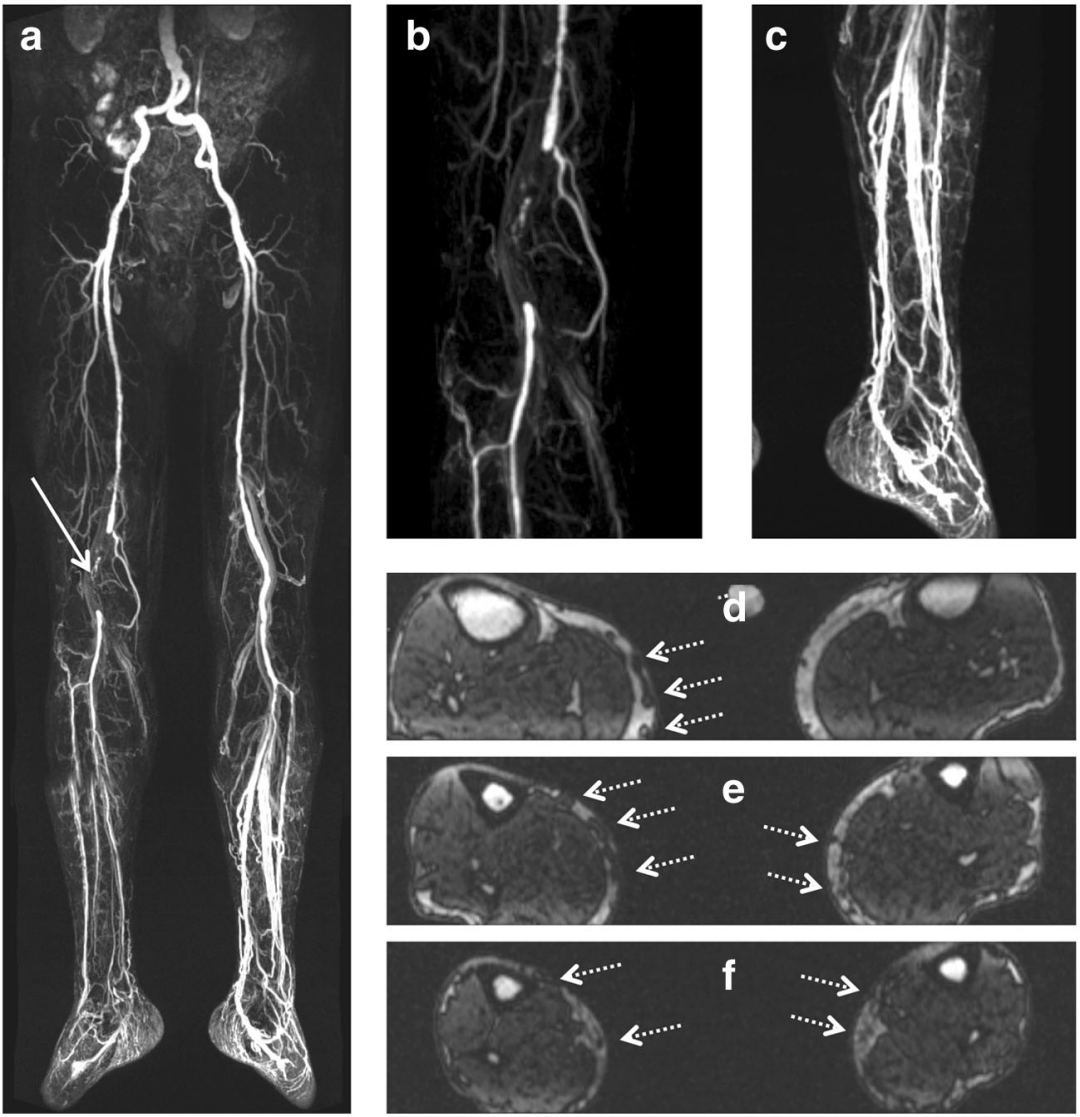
Run-off MR angiography in a 77-year-old male patient with known stage IIb peripheral artery disease. Subtracted images post contrast show occlusion of the right popliteal artery (arrow in a, magnified in b). There is substantial dilatation of the deep venous system particularly in the left lower leg (a, magnified in c). T1-weighted pre-contrast images show varicosis of the superficial veins of both lower legs medially (dotted arrows in d–f)
